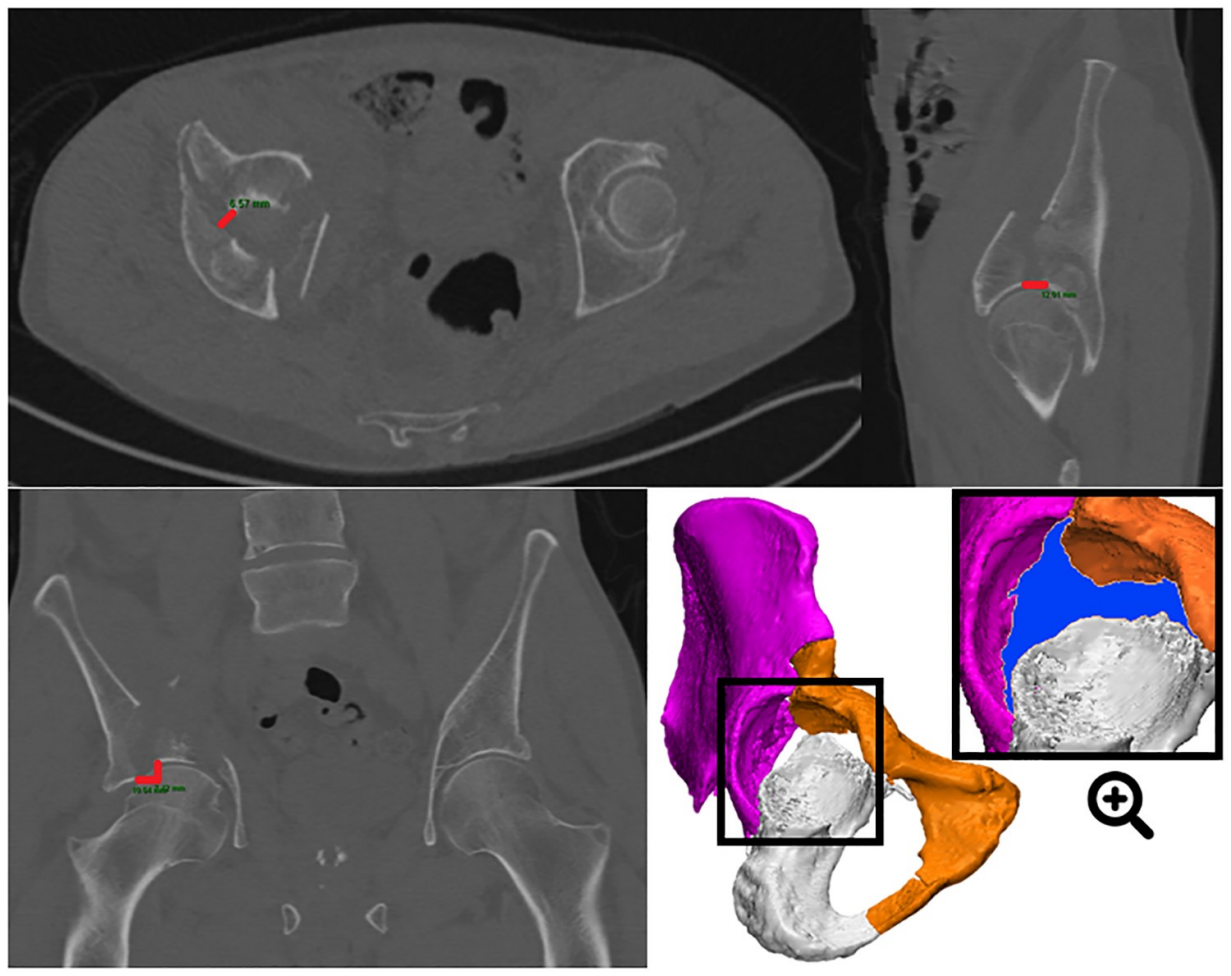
Case example showing both 2DCT and 3DCT measurements of an anterior column and posterior hemitransverse type acetabular fracture with multiple fracture lines going in different directions. 2DCT measurements were performed on the preoperative axial (step-off 0 mm; gap 7 mm), coronal (step-off 7 mm; gap 11 mm) and sagittal (step-off 0 mm; gap 12 mm) CT slices, resulting in a maximum 2D step-off of 7 mm and gap of 12 mm. The direction of measuring the maximum gap is arbitrary, particularly on the axial CT slice. 3DCT measurements, performed on a preoperative 3D model, resulted in a larger maximum gap (2D vs. 3D; 12 vs. 16) and step-off (2D vs. 3D; 7 vs. 16). The total gap area, defined as the surface area between all fracture lines, is marked in blue on the preoperative 3D model.
